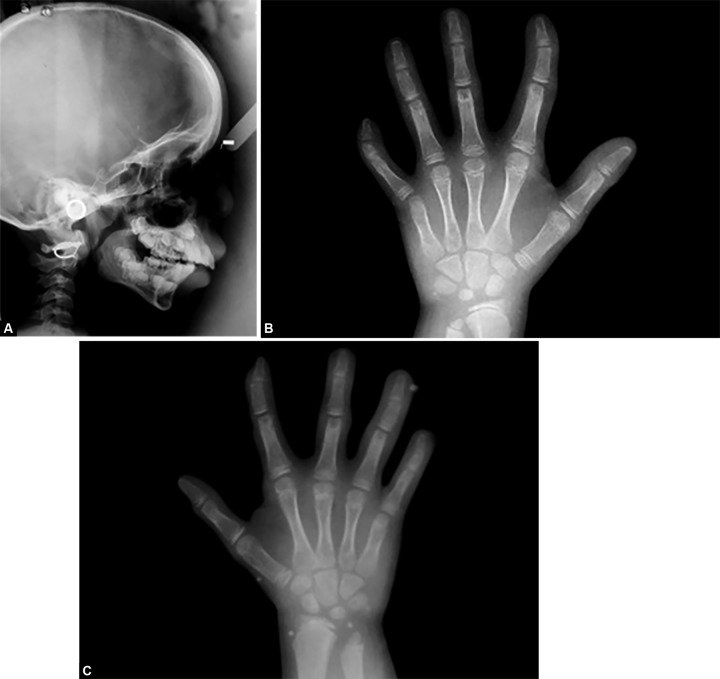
(A) Lateral cephalograph revealing midfacial hypoplasia and mandibular retrognathism, (B) Handwrist radiograph of the right hand showing clinodactyly of the second and fifth finger and (C) Handwrist radiograph of the left hand showing clinodactyly of the second and fifth finger
